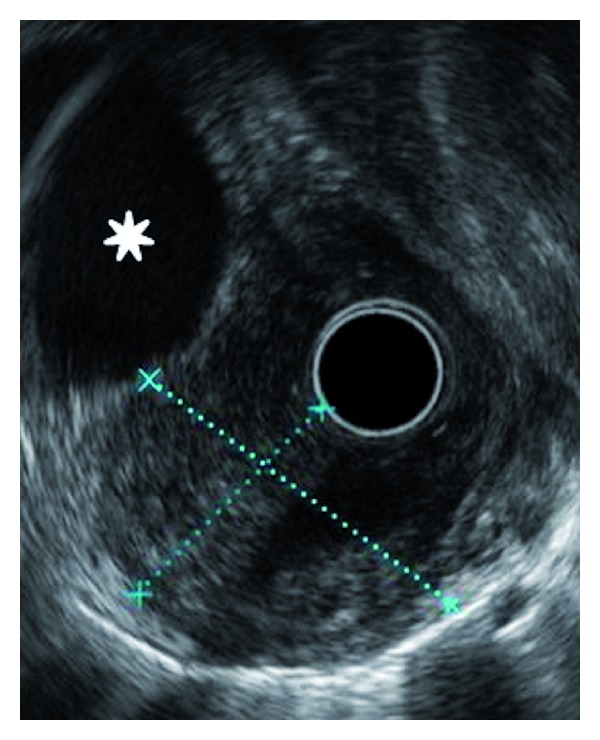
Endoscopic sonographic image showing well-circumscribed hypoechoic structure in the distal esophagus (blue calipers). There is broad abutment with the aorta (small star).
